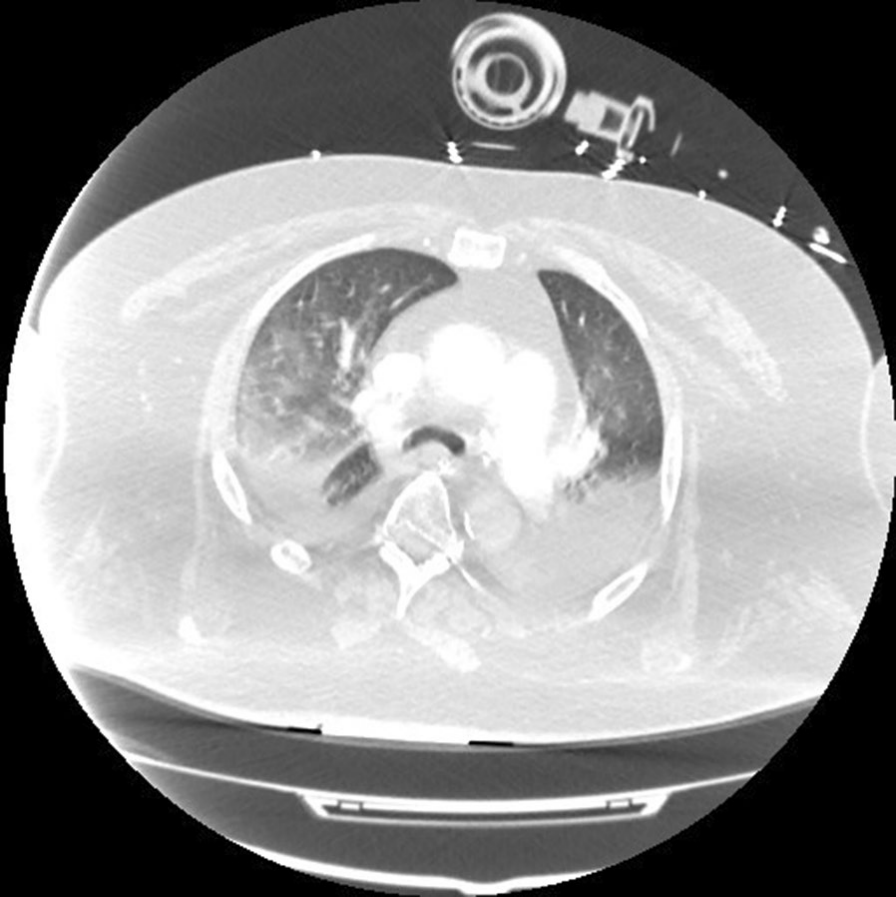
Computed tomography chest transverse view in June 2020
